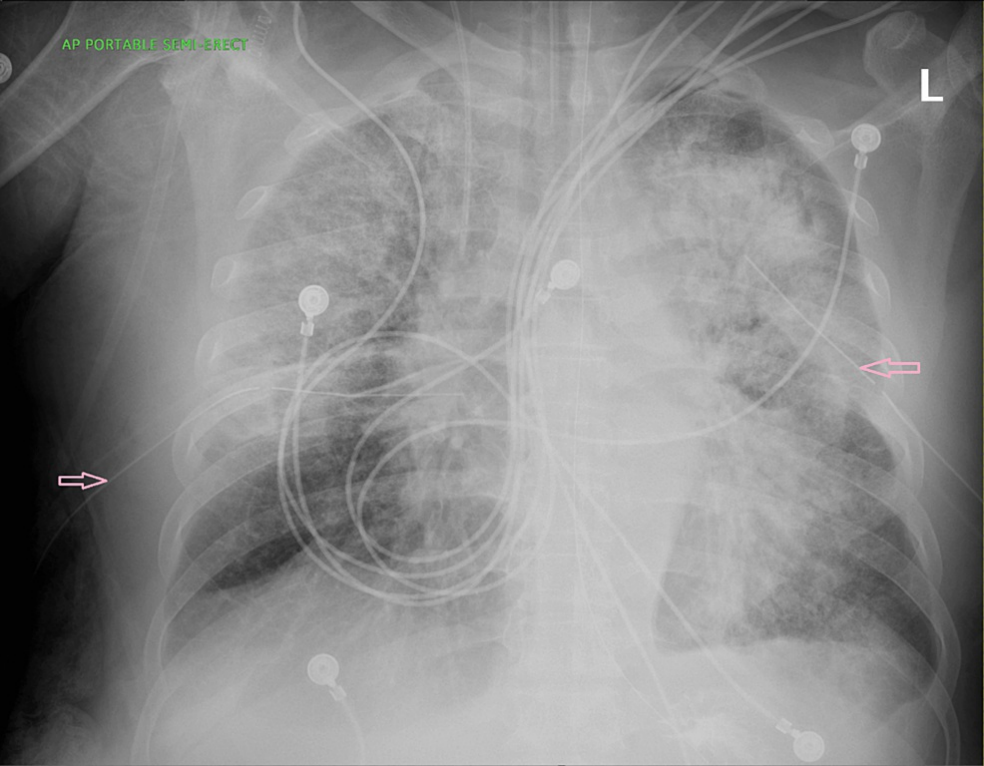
Chest x-ray showing bilateral chest tubes in place
